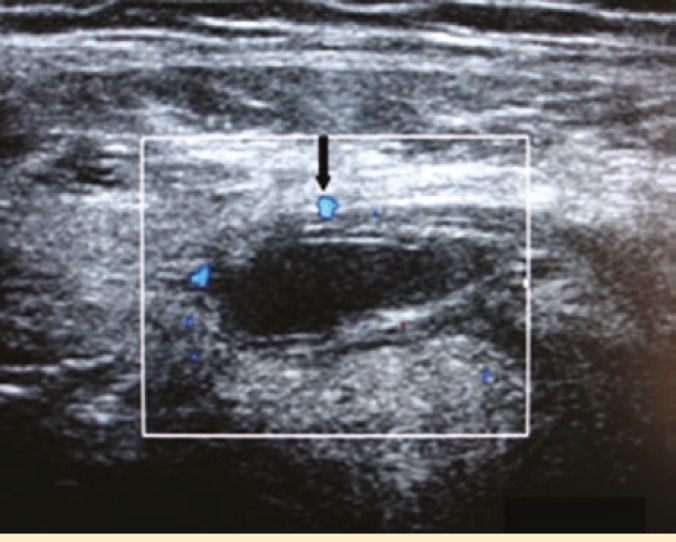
Ultrasound scan showing hypoechoic lesion lateral to the rectus abdominis
